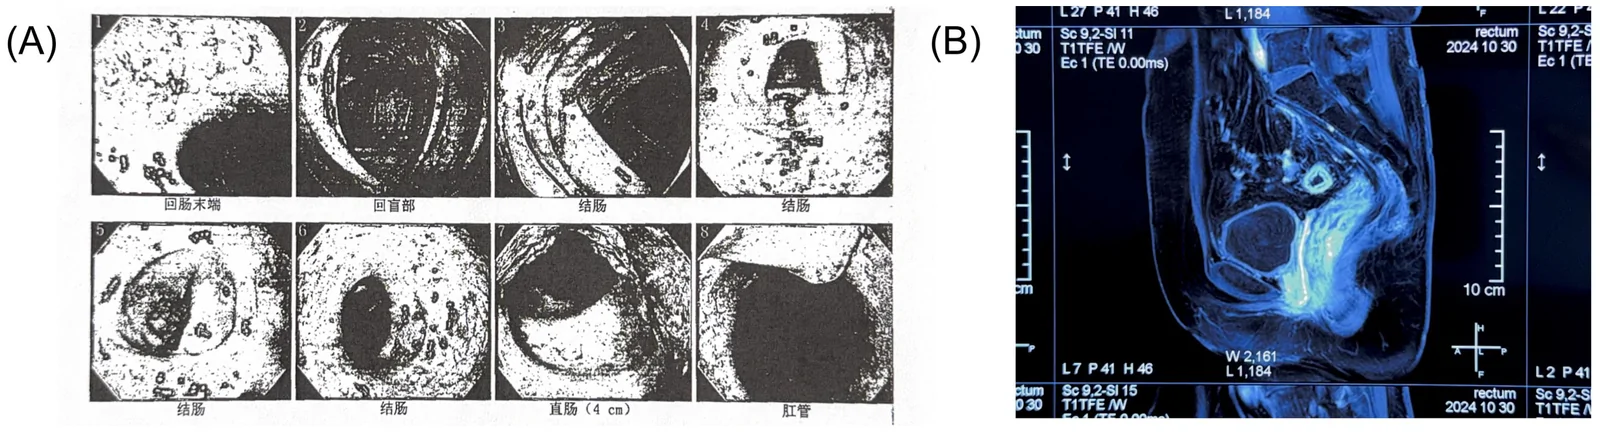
The patient underwent a series of treatments at an external hospital and was subsequently evaluated for their condition. (A) Colonoscopy revealed an ulcerated mass located in the rectum approximately 4 cm from the anal verge; the base of the ulcer was deep and covered with copious fecal matter, the ulcer margin exhibited irregular elevation, the mass had a friable texture that caused bleeding upon palpation, and the intestinal lumen at the site of the mass demonstrated eccentric stenosis. (B) MRI imaging showed that the size of the rectal mass had decreased from the initial measurement of 5.7 cm to 3.6 cm, but the mass remained present.
